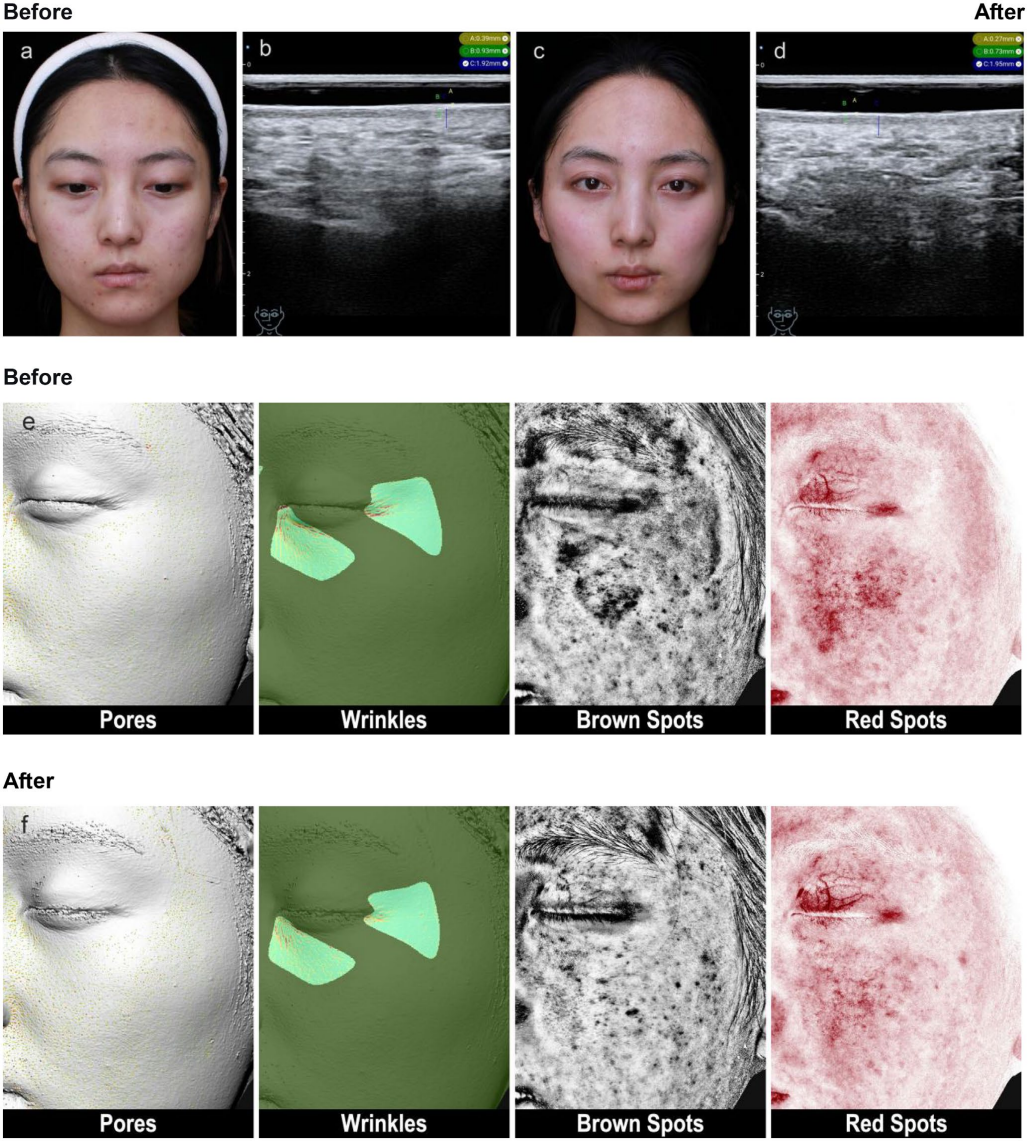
Typical case 1. “26‐year‐old female, relatively dull and sallow complexion, slight redness in the mid‐face apple muscle area, thin stratum corneum, some fine lines under the eyes.” (a) “Clinical image before PRP treatment.” (b) “High‐frequency ultrasound (HFUS) measurement of epidermis, SLEB, and dermal thickness in the nasolabial fold area.” (c) “Clinical image four weeks after the third PRP treatment.” (d) “HFUS measurement of skin thickness in the nasolabial fold area four weeks after the third PRP treatment.” (e, f) “Skin analyzer images of Pores, Wrinkles, Brown Spots, Red Spots before and four weeks after the third PRP treatment.”
